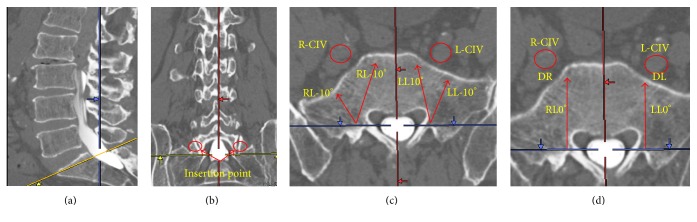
(a) Sagittal computed tomography image. The yellow line indicates the transarticular surface screw trajectory. (b) The red circle indicates the insertion point of the TASS in the coronal view. (c) R-CIV: right common iliac vein; L-CIV: left common iliac vein; RL10: trajectory 10° lateral to the insertion point; RL-10: trajectory 10° medial to the insertion point on the right side. LL10 and LL-10 are consistent with the same definitions on the left side. (d) RL0 trajectory straight and perpendicular to a horizontal line from the insertion point. DR and DL indicate the distance between the nearest wall of the CIV and the anterior wall of the S1 vertebra on the right and left side, respectively.
